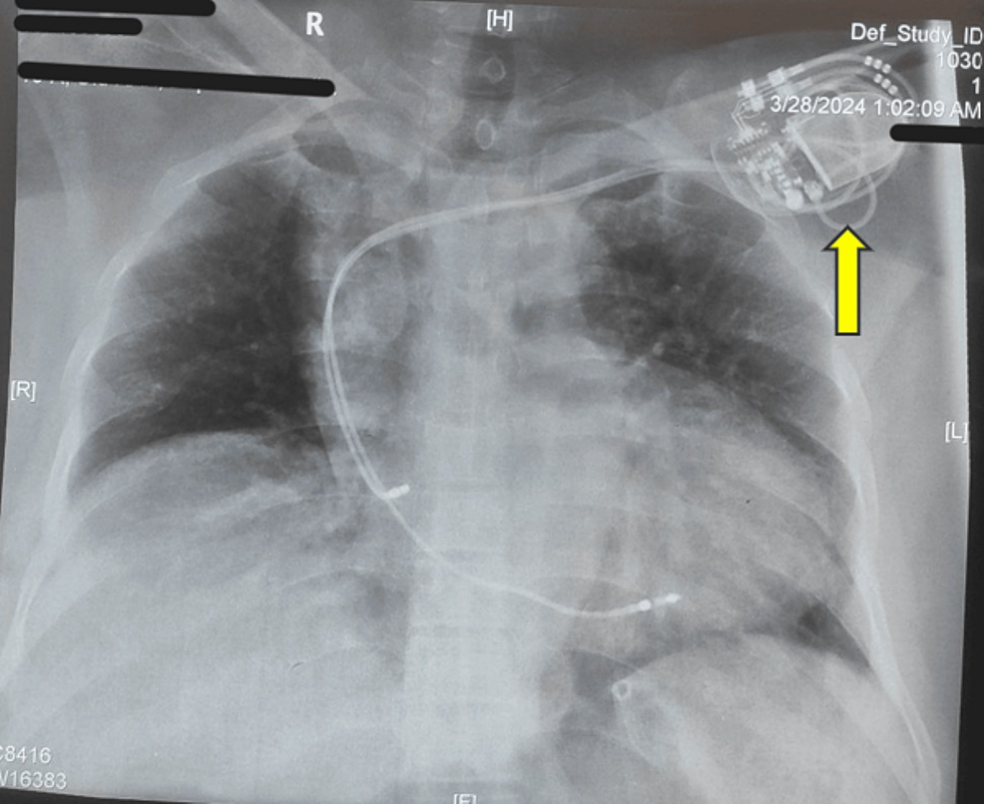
Chest X-ray of the patient showing cardiomegaly and pacemaker in situ in the left pectoral region (yellow arrow)
